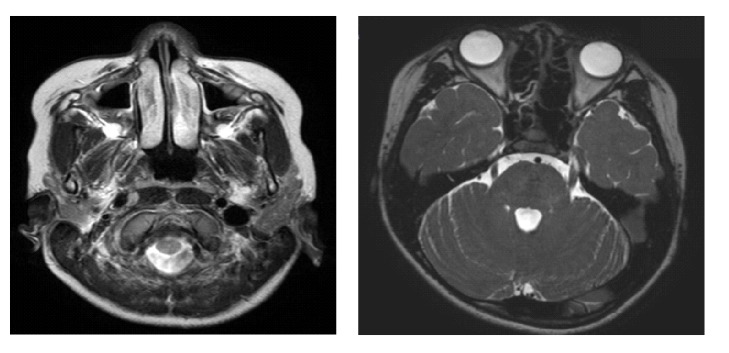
Head imaging study. Head computed tomography showed mucous membrane swelling and fluid collection in both maximal sinuses (left). Brain magnetic resonance image. Vascular compression of the trigeminal nerve was not observed (right).
